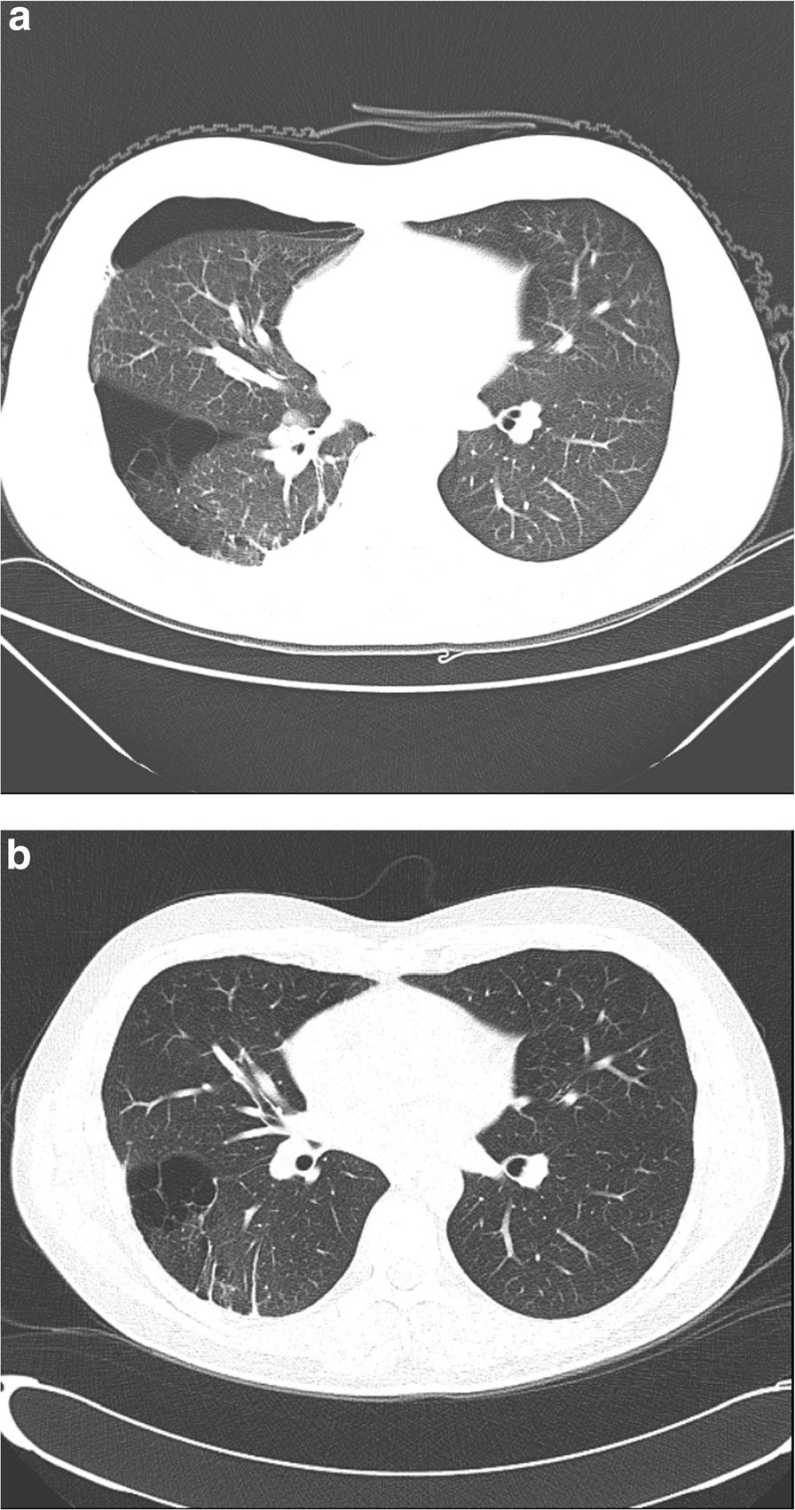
Thoracic CT of case 2. a. CT image of pneumothorax with a cluster of lung cysts in the right lower lobe. b. Shows a lung cyst located subpleurally in the right lower lobe, abutting the main fissure as shown after resolving of the pneumothorax.
